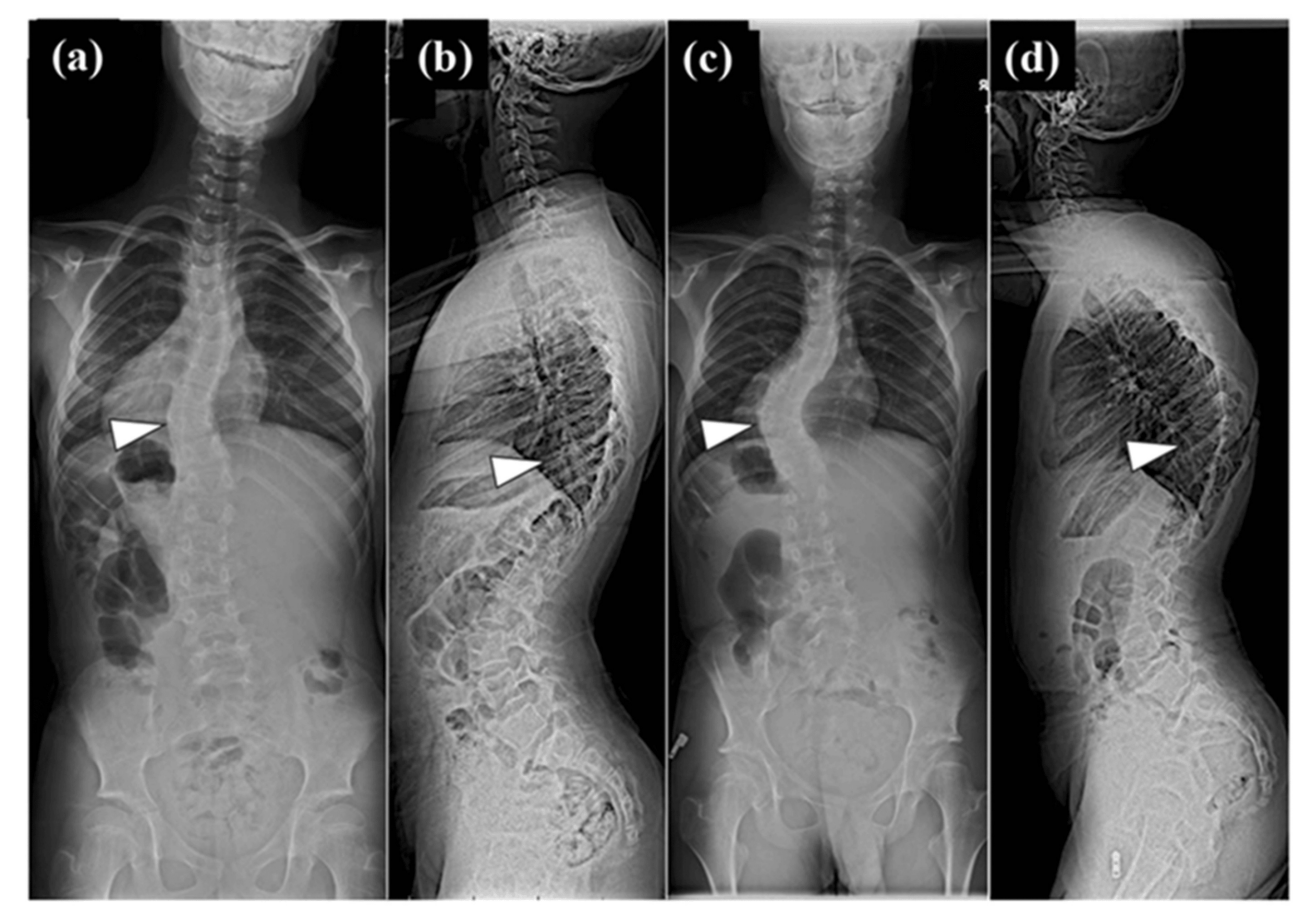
Coronal and sagittal standing full-spine radiograph taken 1.5 years before and at the time of surgery. Coronal standing full-spine radiograph taken 1.5 years before surgery (a) and at the time of surgery (b) showed the Cobb angle of the scoliotic major curve from T9 to T11 was 35 degrees and 60 degrees, respectively. Sagittal standing full-spine radiograph taken 1.5 years before surgery (c) and at the time of surgery (d) showed the Cobb angle of the local kyphosis from T8 to T11 was 35 degrees and 60 degrees, respectively. The white triangle indicated the T10 vertebra in each image.
